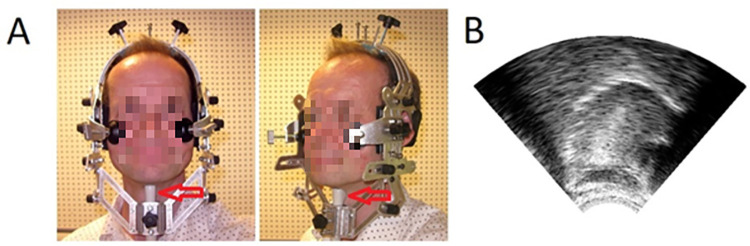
Ultrasound apparatus and example image. Panel A shows the stabilization headset for keeping the probe in place under the chin (see red arrow). Panel B shows an example of the fan-shaped ultrasound image with the tongue in mid-sagittal plane, as rendered by the ultrasound probe.
